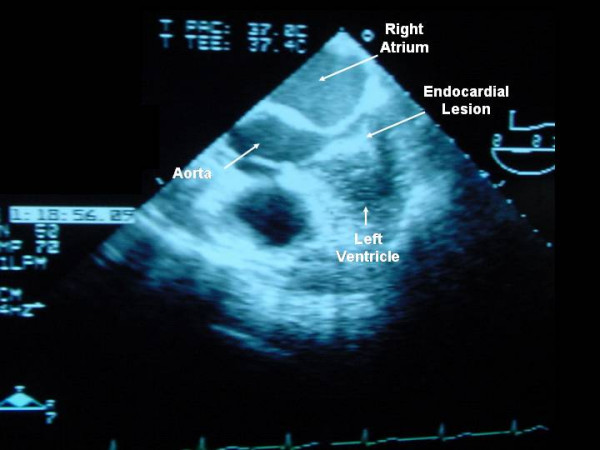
A 2-dimensional transesophageal long-axis echocardiogram showing a mobile, pediculated echodense image adjacent to the lower intraventricular septum.
